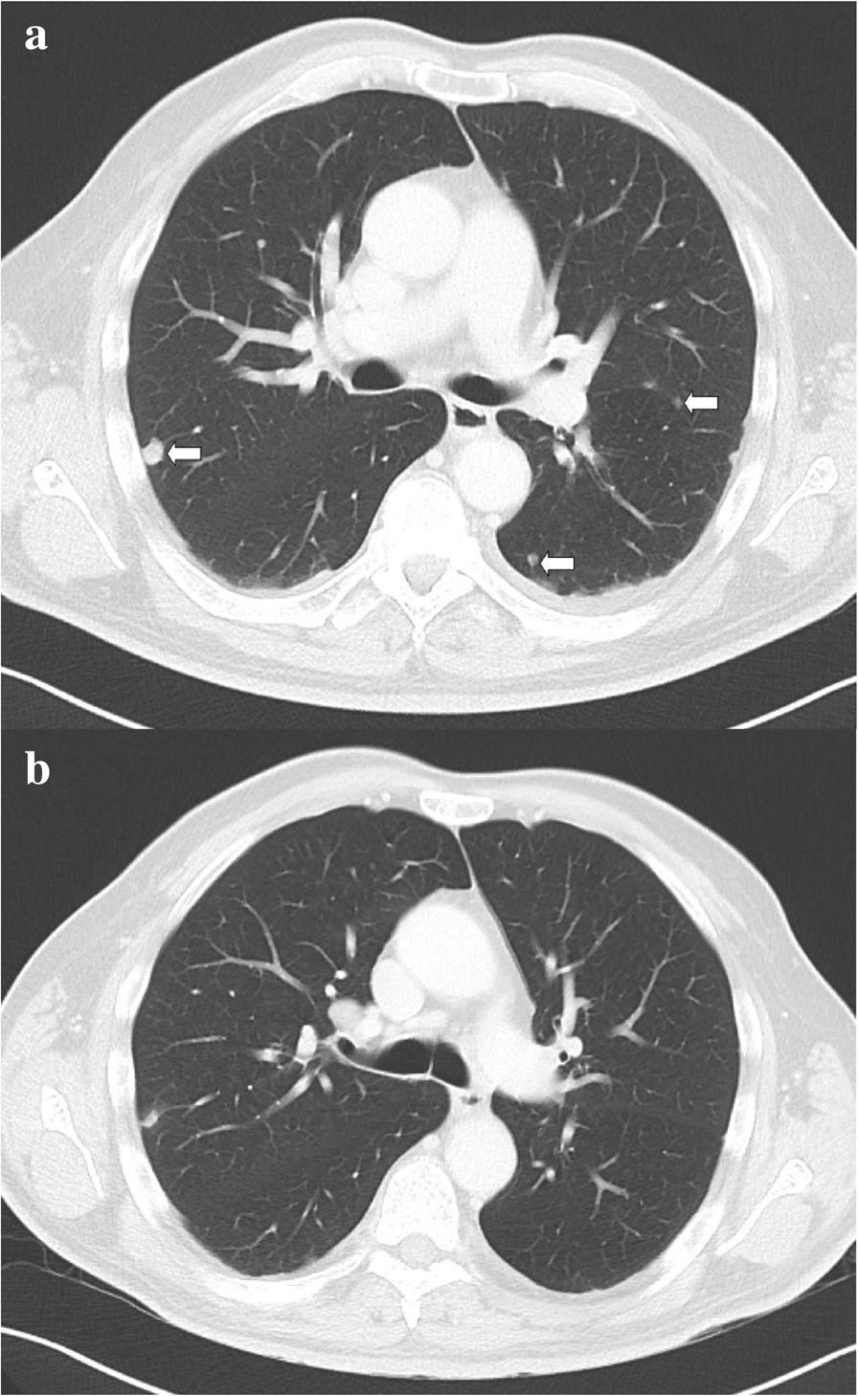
Axial contrast-enhanced computed tomography scans of the thorax showing tumor regression (see arrows) April 2016 (a) and August 2016 (b)
